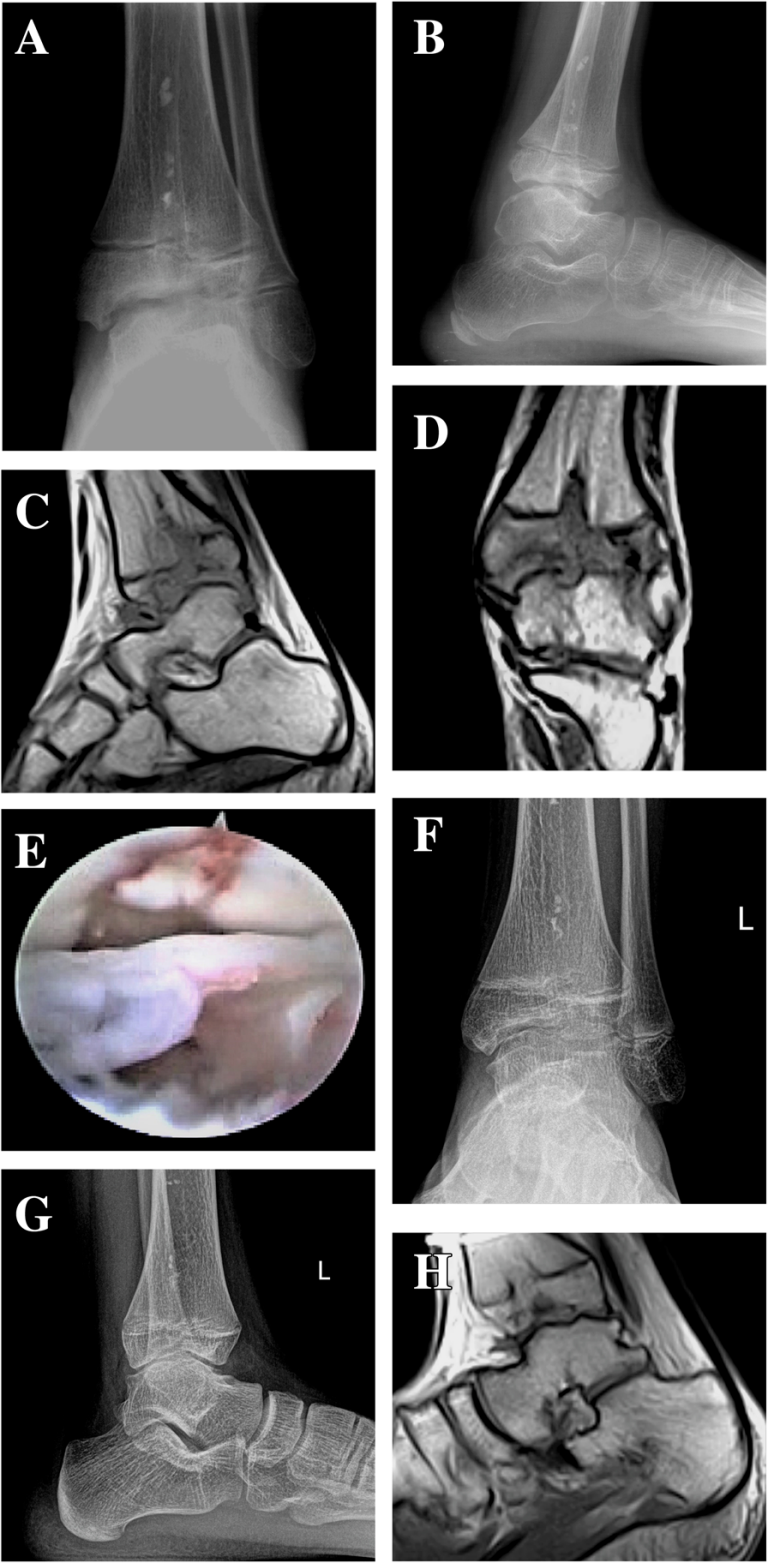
a Preoperative radiograph suggesting unsmooth ankle joint space and epiphyseal injury of the tibia (anteroposterior view). b Preoperative radiograph suggesting unsmooth ankle joint space (lateral view). c Preoperative MRI suggesting ankle joint damage and hyperplasia of synovial tissue (sagittal view). d Preoperative MRI suggesting ankle joint damage and hyperplasia of synovial tissue (coronal view). e Articular cartilage defects, massive synovial hyperplasia, and scar tissue formation seen during arthroscopy. f Radiograph 5 years postoperatively suggesting that the ankle joint is not smooth, but the epiphyseal injury of the tibia is better than before surgery (anteroposterior view). g Radiograph 5 years postoperatively suggesting that the ankle joint is not smooth but is better than before surgery (lateral view). h MRI 5 years postoperatively suggesting that the ankle joint is not smooth (sagittal view)
